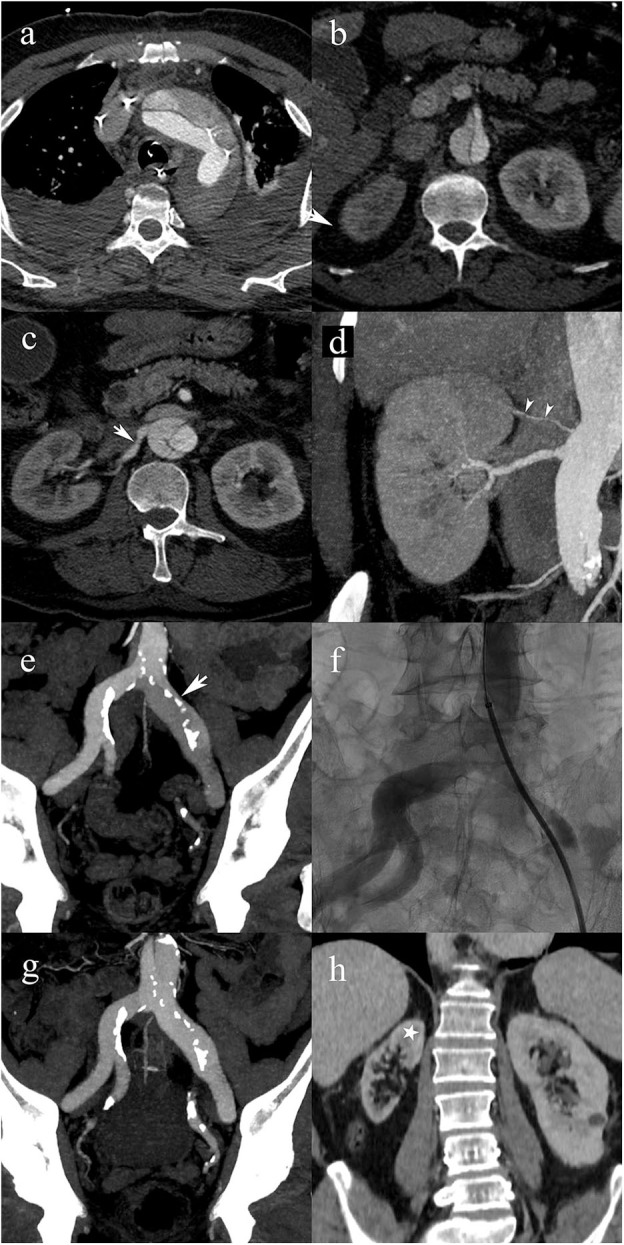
A 63-year-old male patient with type A2 AAD. Patent false lumen (a), without compromise of the superior mesenteric artery (b); post-ostial dissection of the right renal artery (white arrow) (c); post-ostial stenosis of the right renal artery resulting in a static MPS. Note the accessory renal artery supplying the superior pole of the right kidney (white arrowheads) (d). Extension into the left common iliac artery with compression of the true lumen (arrow) resulting in a static MPS of the left lower limb (e); transcatheter angiography shows a significant stenosis of the true lumen in the left common iliac artery (f); follow-up CTA shows that the iliac artery stenosis disappeared following endovascular fenestration procedure (g); 5-year follow-up CTA shows right renal atrophy due to untreated static MPS. Cortical thinning spares the upper pole (star) because of the patent accessory renal artery.
